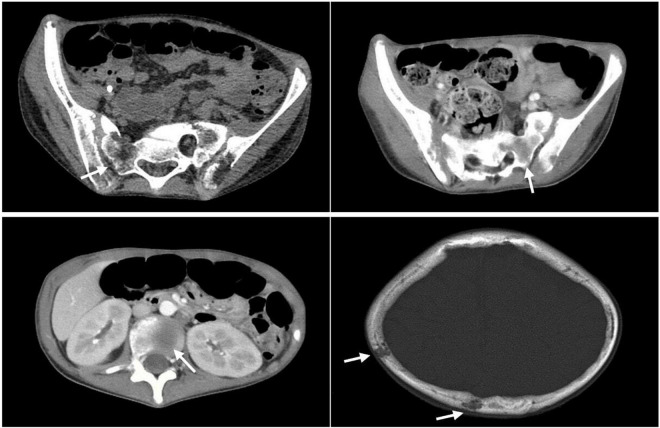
Computed tomography of skull and pelvis showing multiple osteolytic lesions.
